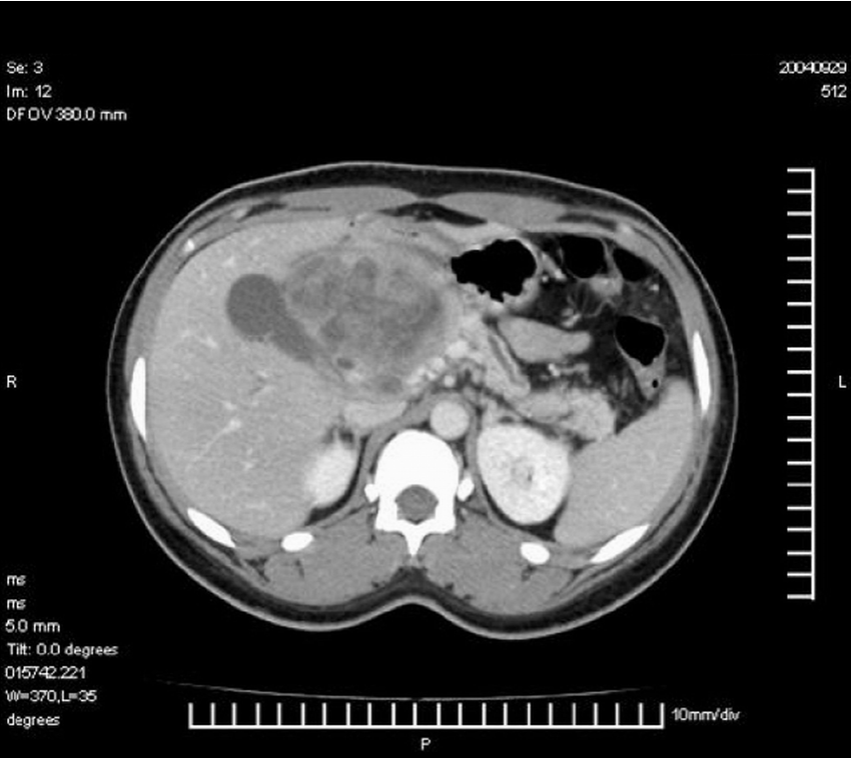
Contrast enhanced CT scan of the abdomen demonstrating a large inhomogenous pancreatic tumour.
